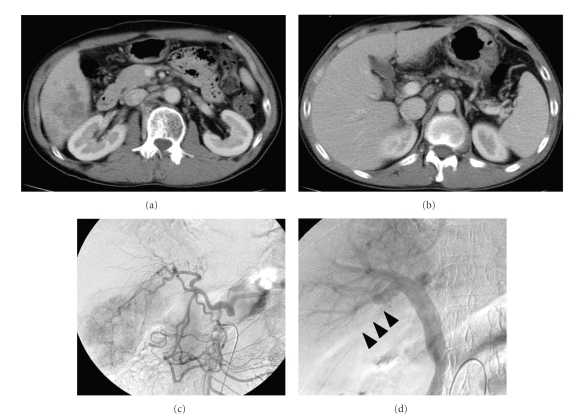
(a) A Computed tomography (CT) showed a peripherally enhanced low density mass 7.5 cm in diameter, which located in segment 6 in the right lobe of the liver. (b) Tumor was accompanied with tumor thrombus to the posterior branch of portal vein. (c) Celiac angiography showed this lesion to be hypervascular. (d) Portal vein tumor thrombus in posterior segment was observed in portal phase of SMA angiography.
